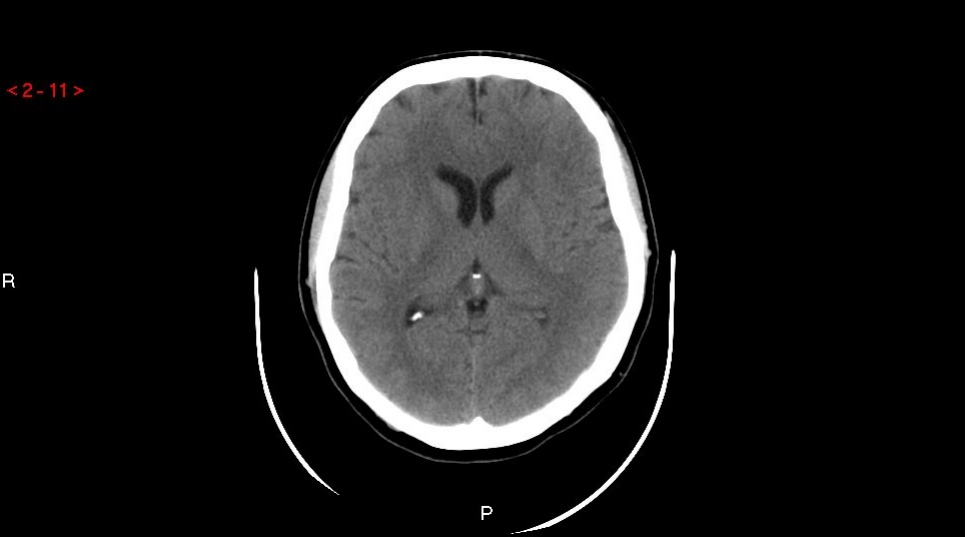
Brain Computed tomography performed during the first recovery.
